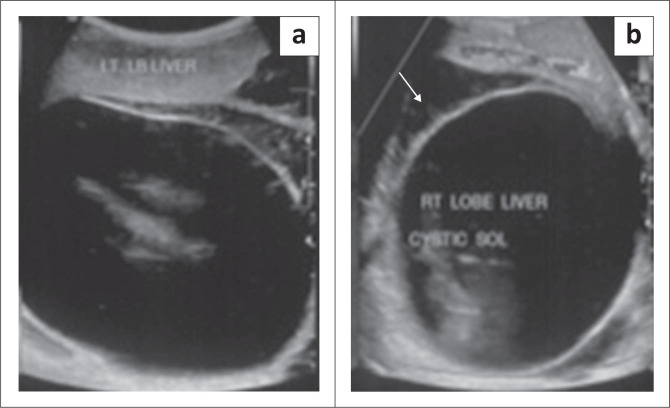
A 1-year-old female with a mesenchymal hamartoma presented with an abdominal mass. Transverse greyscale ultrasound abdomen (a and b) revealed a large, unilocular, cystic mass in the left lobe of liver with adjacent fluid (arrow in b) suggestive of localised rupture.
